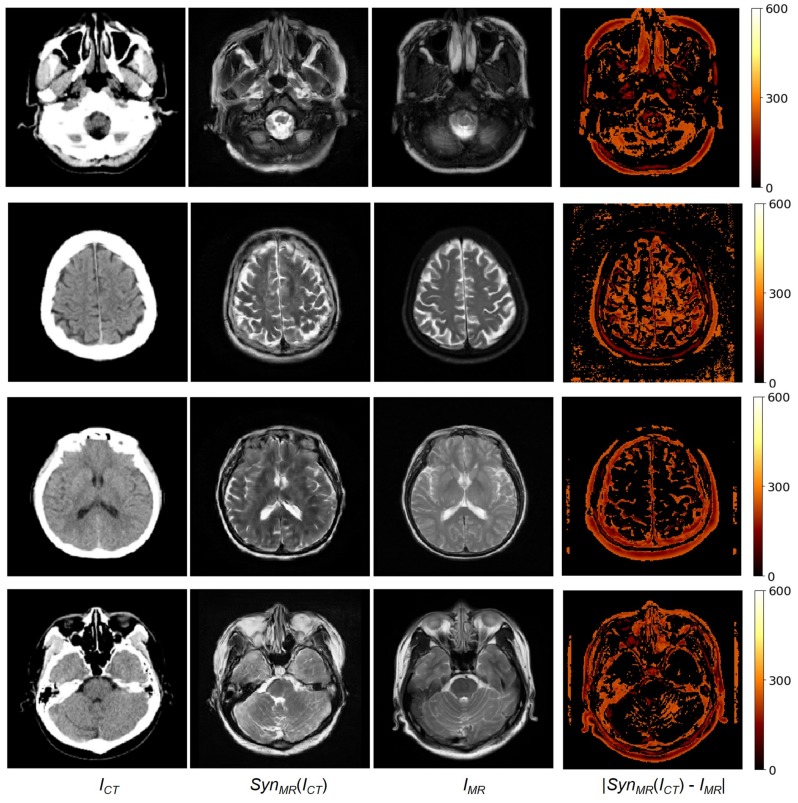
From (left) to (right): Input CT, synthesized MR, reference MR, and absolute error between reference and synthesized MR images.
